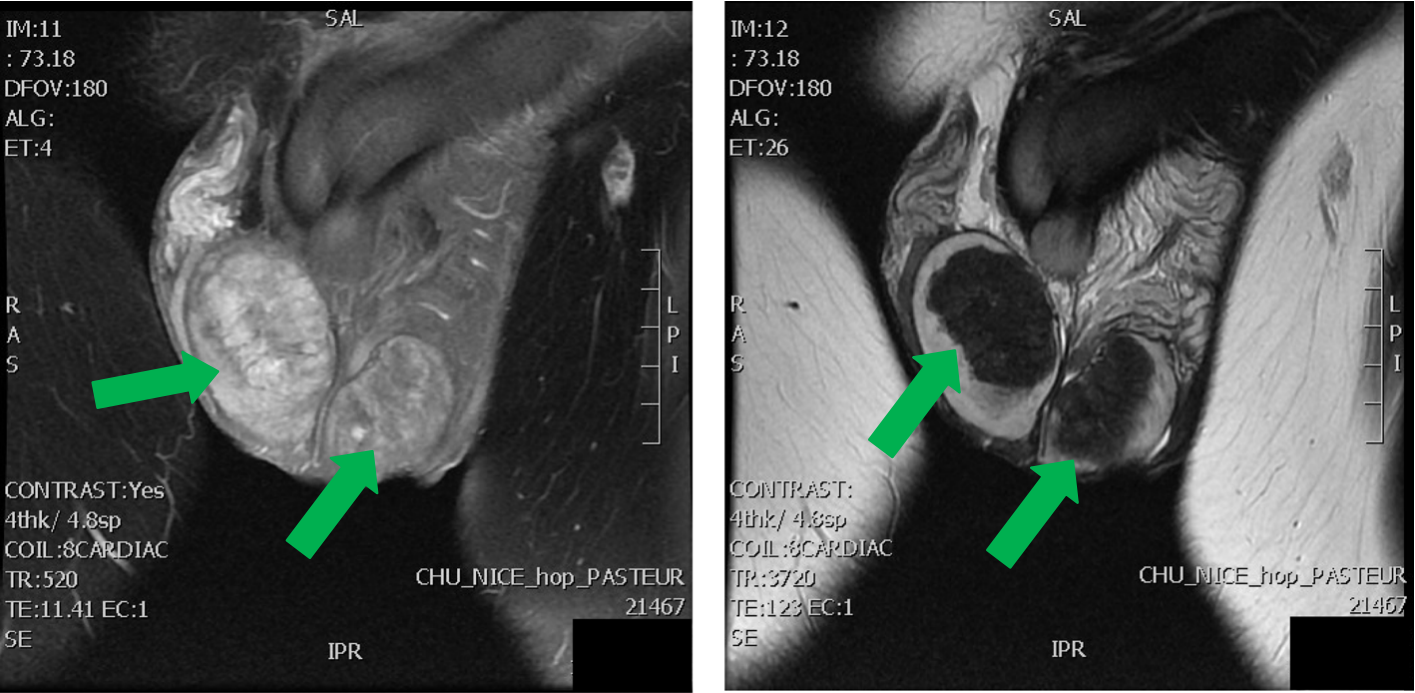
Scrotal MRI of the testis from second case Legend. Arrows indicate Testicular Adrenal Rest Tumors (TART)
